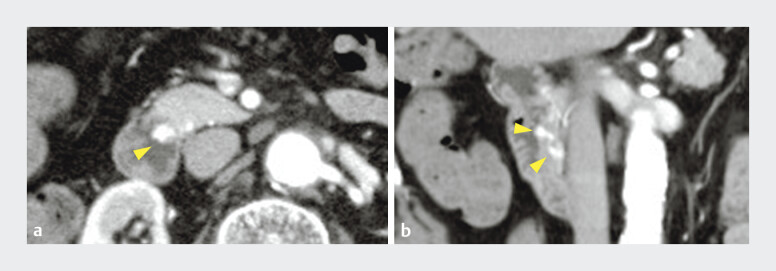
Contrast-enhanced computed tomography revealed a vascular lesion in the duodenal papilla (arrowhead), suspected to be an aneurysm, which showed enhancement in the early phase (
